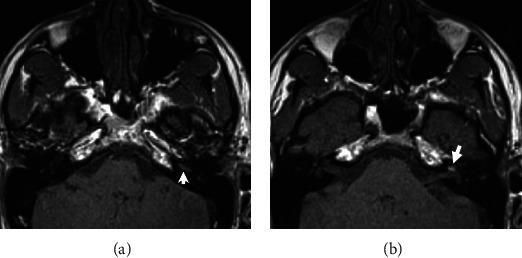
Magnetic resonance imaging of the temporal bone: axial T1-weighted slice before gadolinium injection showing hyperintensity of cochlear basal (a) and second turns (b) of the left ear with a normal signal in the right ear.
